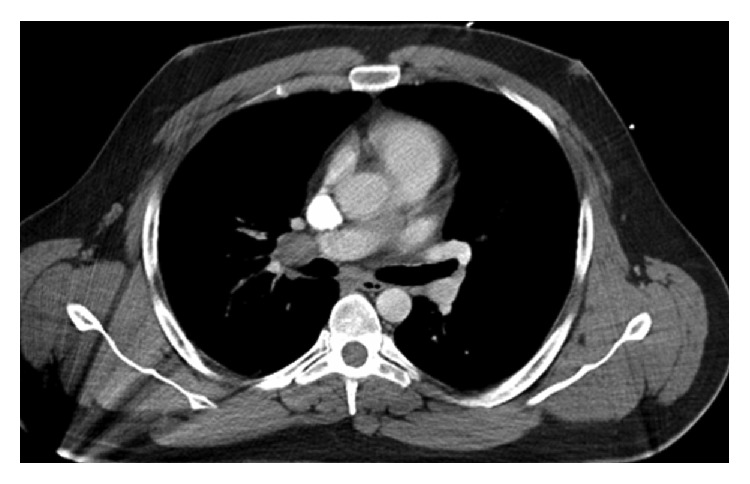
High resolution CT scan of hilar lymphadenopathy with pulmonary vein compression.
